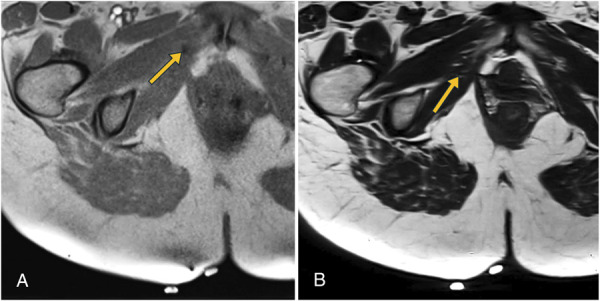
Identification of anchor on preprocedural imaging. A. The anchor is seen as a signal void (*yellow arrow*) on three-dimensional gradient echo (GRE) T1-weighted imaging. B. The anchor seen as a low-signal structure on T2-weighted turbo spin echo imaging (*yellow arrow*), corresponding to the known appearance of the anchor and the location identified on GRE.
